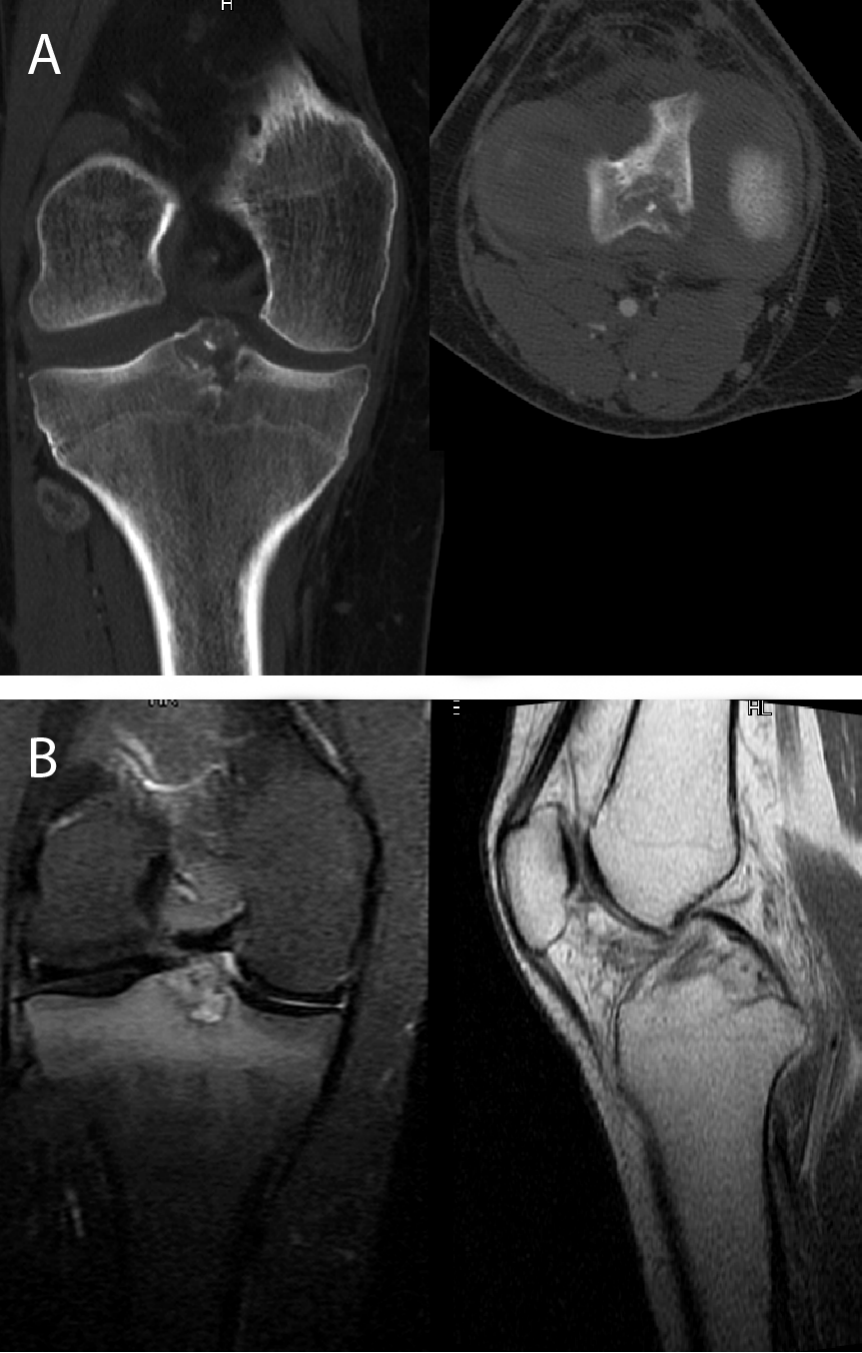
A-B CT and MRI scan showing an osteolytic lesion in the spines area.
